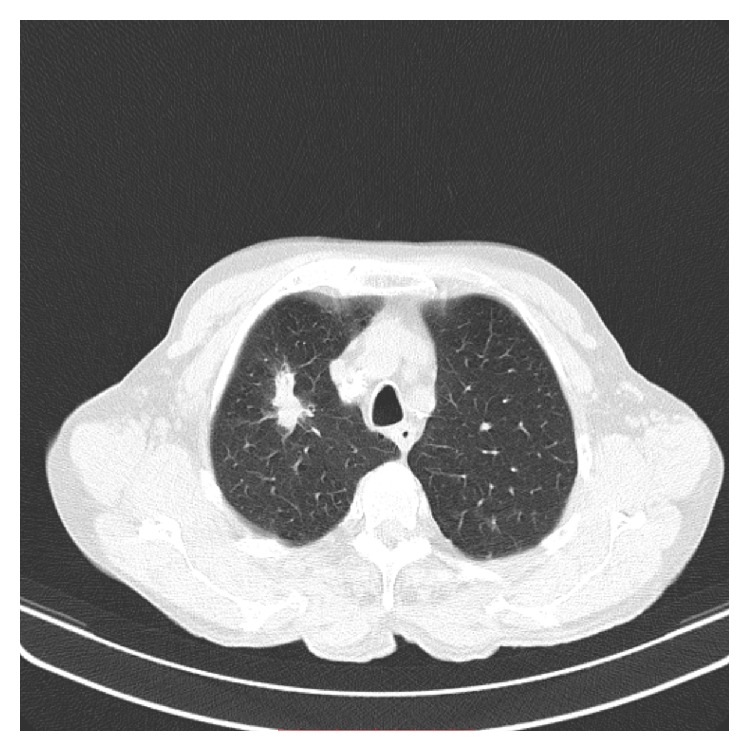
CT-scan of the chest showing an irregular elongated shadow in apical segment of right upper lobe.
